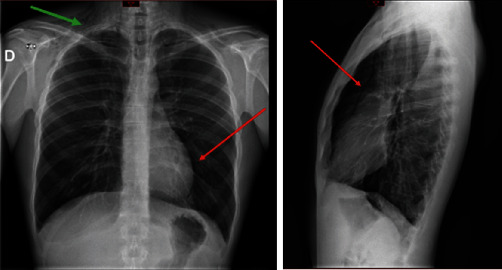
Chest radiograph of the face and profile. Supraclavicular subcutaneous emphysema (green arrow) and pneumomediastinum (red arrows; retrosternal radiolucency following the contour of the heart and aorta) are observed.
